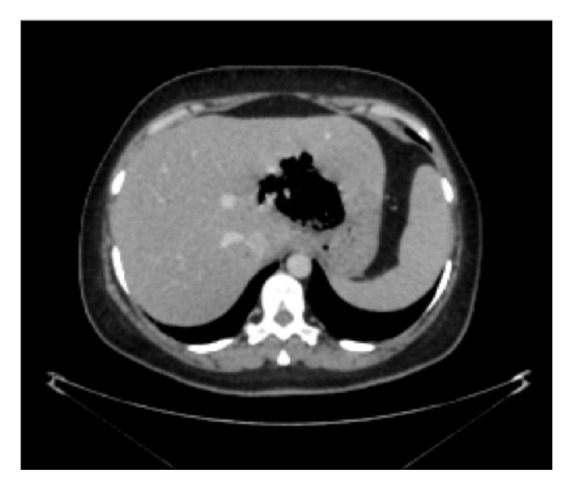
Axial abdominal CT scan with oral and IV contrast. Mixed collection containing air and debris spreading at the posterior aspect of the left lobe of the liver mainly in segments II and III, limited laterally by the left portal vein and extending medially towered the lesser curvature of the stomach associated with moderate intra- and extrahepatic biliary dilation. Sharply marginated left borders are noted.
